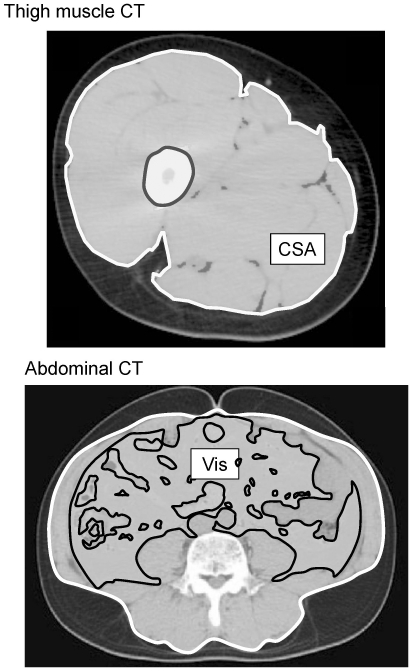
Thigh muscle CT (top) at the mid-thigh level and abdominal CT (bottom) at umbilicus level. Thigh muscle cross-sectional area (CAS) and visceral fat area (Vis) were obtained.
